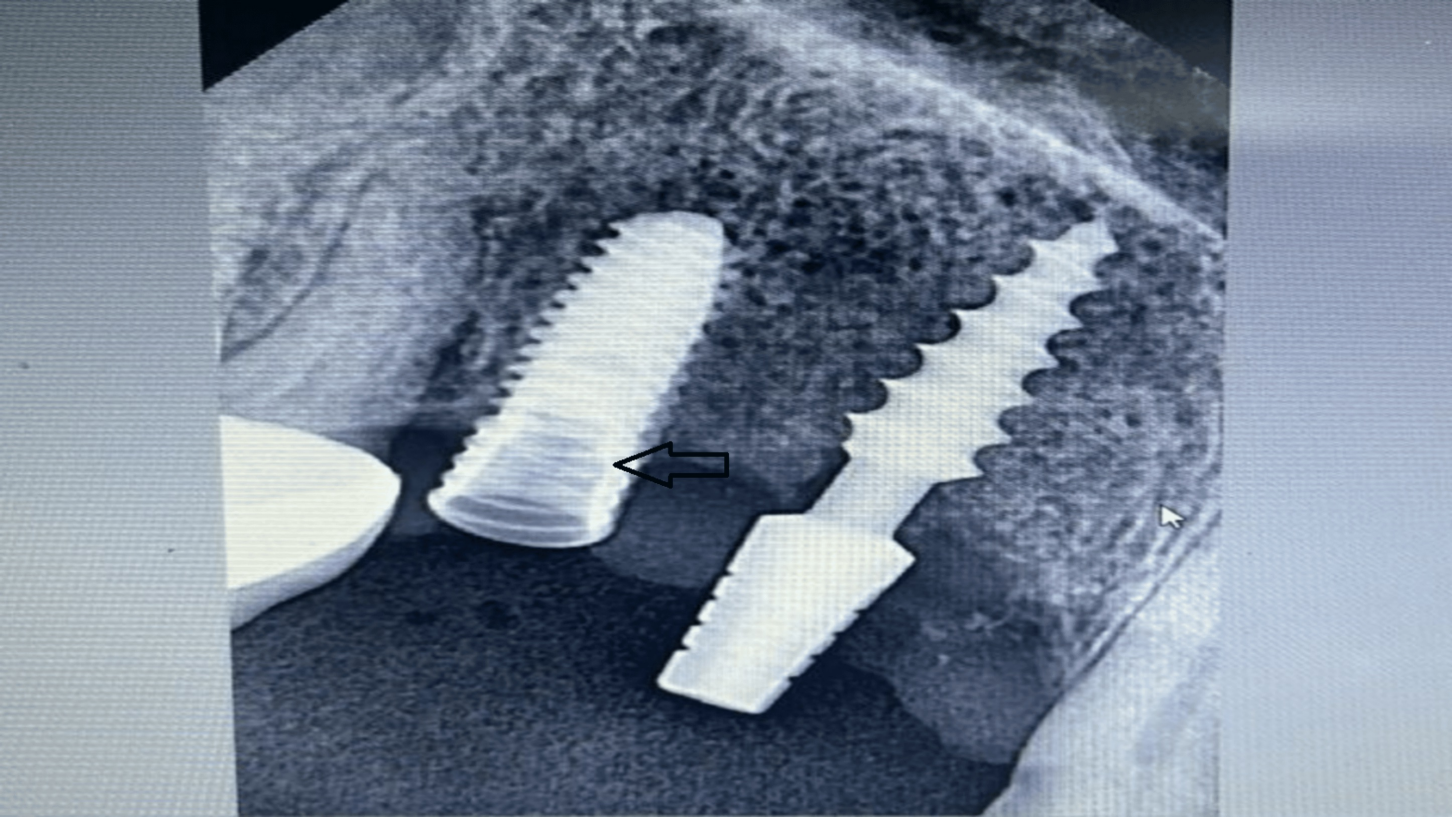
Radiographic view showing fractured abutment screw
